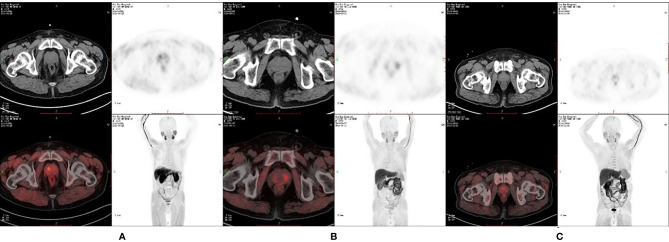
^11^C-choline positron emission tomography (PET) image of patients with different prostate diseases. (A) SUVmax is 5.28, SUVmean is 3.24 and P/M ratio is 8.07 in patients with PCa. (B) SUVmax is 3.04, SUVmean is 2.64 and P/M ratio is 3.50 in patients with benign prostate hyperplasia (BPH). (C) SUVmax is 2.36, SUVmean is 1.67 and P/M ratio is 2.49 in patients with chronic protatitis (CP).
